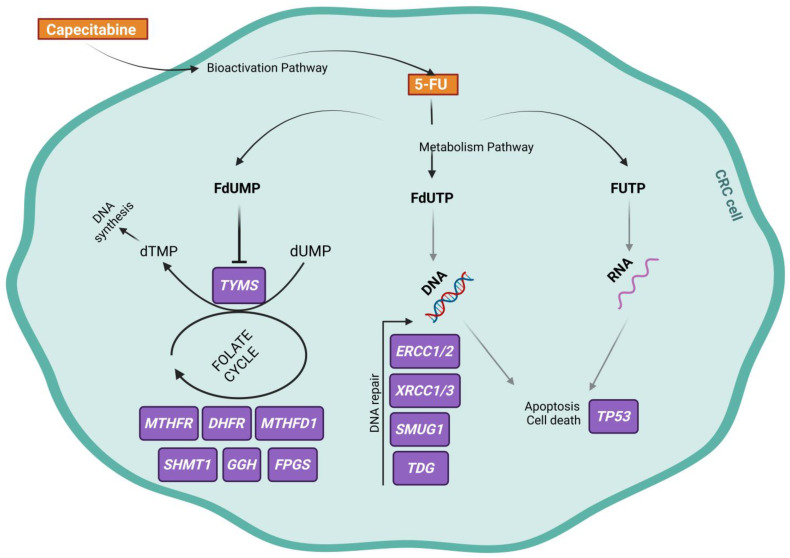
Capecitabine pharmacodynamics pathway. Created with BioRender.com. 5-FU: fluorouracil; CRC: colorectal cancer; dTMP: 2′-deoxythymidine-5′-monophosphate; dUMP: 2′-deoxyridine-5′-monophosphate; DHFR: dihydrofolate reductase; *ERCC1*: ERCC excision repair 1, endonuclease non-catalytic subunit; *ERCC2*: ERCC excision repair 2, TFIIH core complex helicase subunit; *ENOSF1*: enolase superfamily member 1; FdUMP: fluorodeoxyuridine monophosphate; FdUTP: fluorodeoxyuridine triphosphate; FPGS: folylpolyglutamate synthase; FUTP: fluorouridine triphosphate; GGH: gamma-glutamyl hydrolase; MTHFD1: methylenetetrahydrofolate dehydrogenase; *MTHFR*: methylenetetrahydrofolate reductase; SHMT1: serine hydroxy methyltransferase 1; SMUG1: single-strand-selective monofunctional uracil-DNA glycosylase 1; SNP: single-nucleotide polymorphism; TDG: thymine DNA glycosylase; TP53: tumor protein P53; *TYMS*: thymidylate synthetase; *XRCC1*: X-ray repair cross-complementing 1; *XRCC3*: X-ray repair cross-complementing 3.
